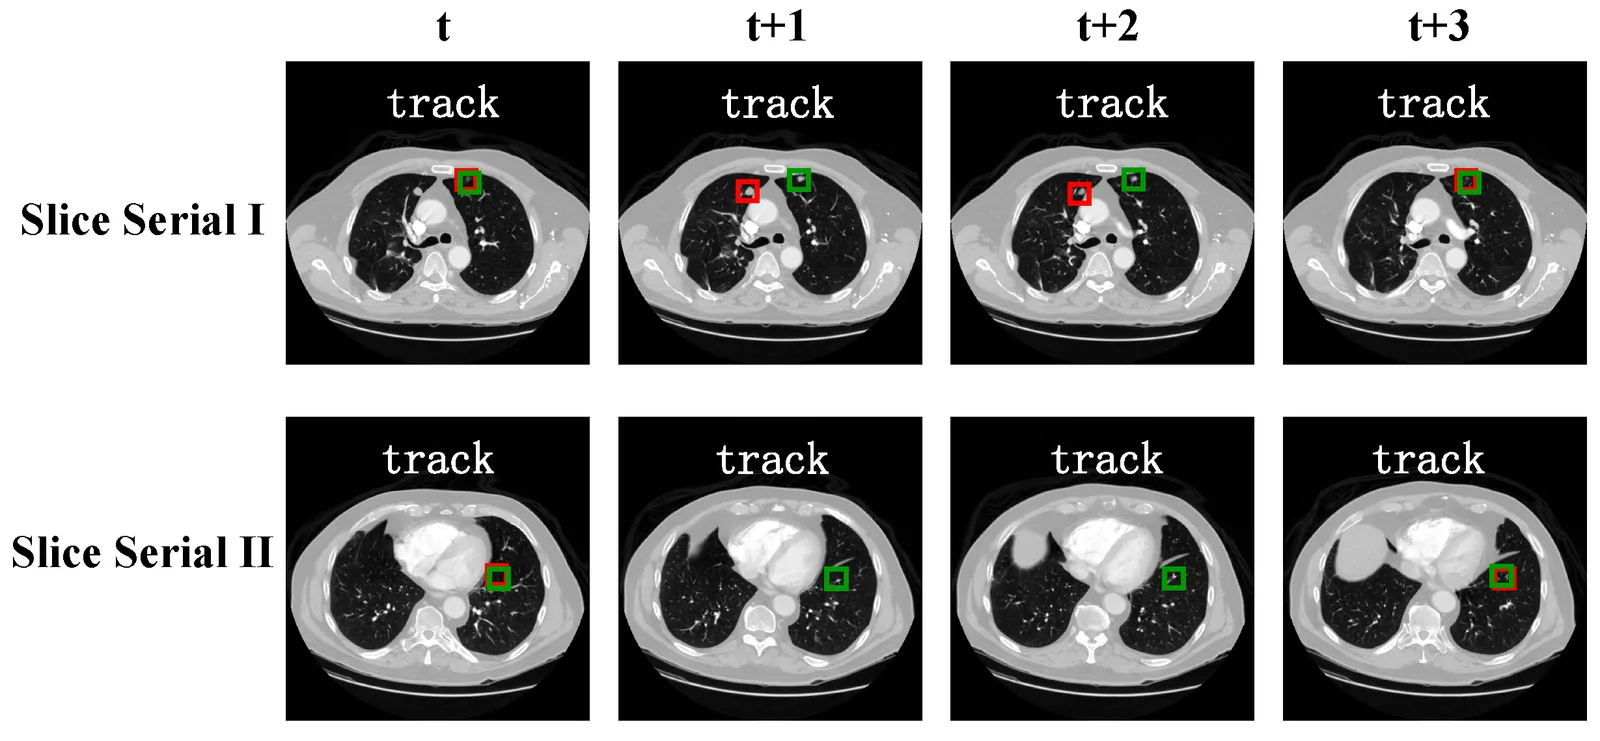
Comparison of CNT algorithm tracking for detected and missed objects. The red boxes denote the raw 2D detection outputs. The green boxes represent the tracked boxes obtained via the CNT algorithm. Row I and Row II each show a sequence of four consecutive CT slices.
